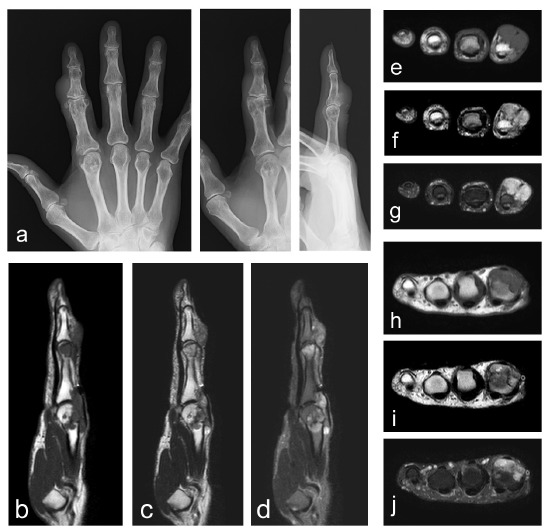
Images of a 65-year-old female with extra-articular tenosynovial chondromatosis. The plain radiographs of the index finger of the right hand show irregularity of the cortex at the distal end of the proximal phalanx and of the second metacarpal bone (a: anteroposterior; left, oblique; middle, lateral; right). MRIs (b-j) show multinodular lesions around the proximal interphalangeal joint (e-g) and metacarpophalangeal joint (h–j) levels with homogeneous low signal intensity on the T1-weighted images (b, e, and h), and heterogeneous low to high signal intensity on the T2-weighted images (c, f, and i) with fat-suppression (d, g and j). Osseous invasion is apparent.
